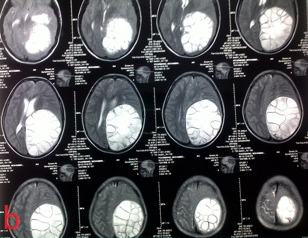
T2‐weighted axial MRI of the brain shows a large cystic left parietooccipital lobe, paraventricular area
